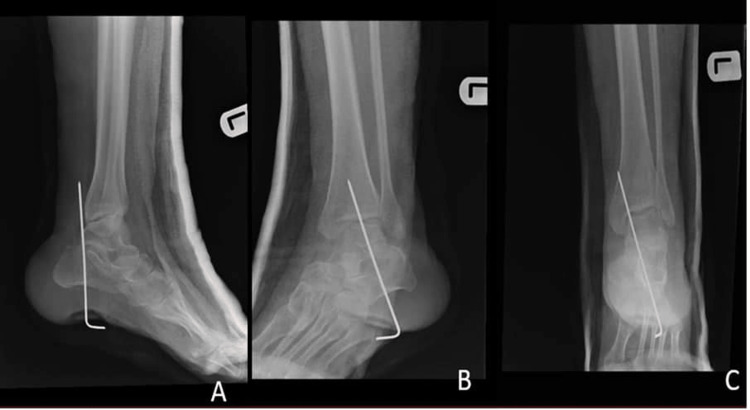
Postoperative X-ray of the left ankle: (A) lateral, (B) oblique, and (C) anteroposterior views
